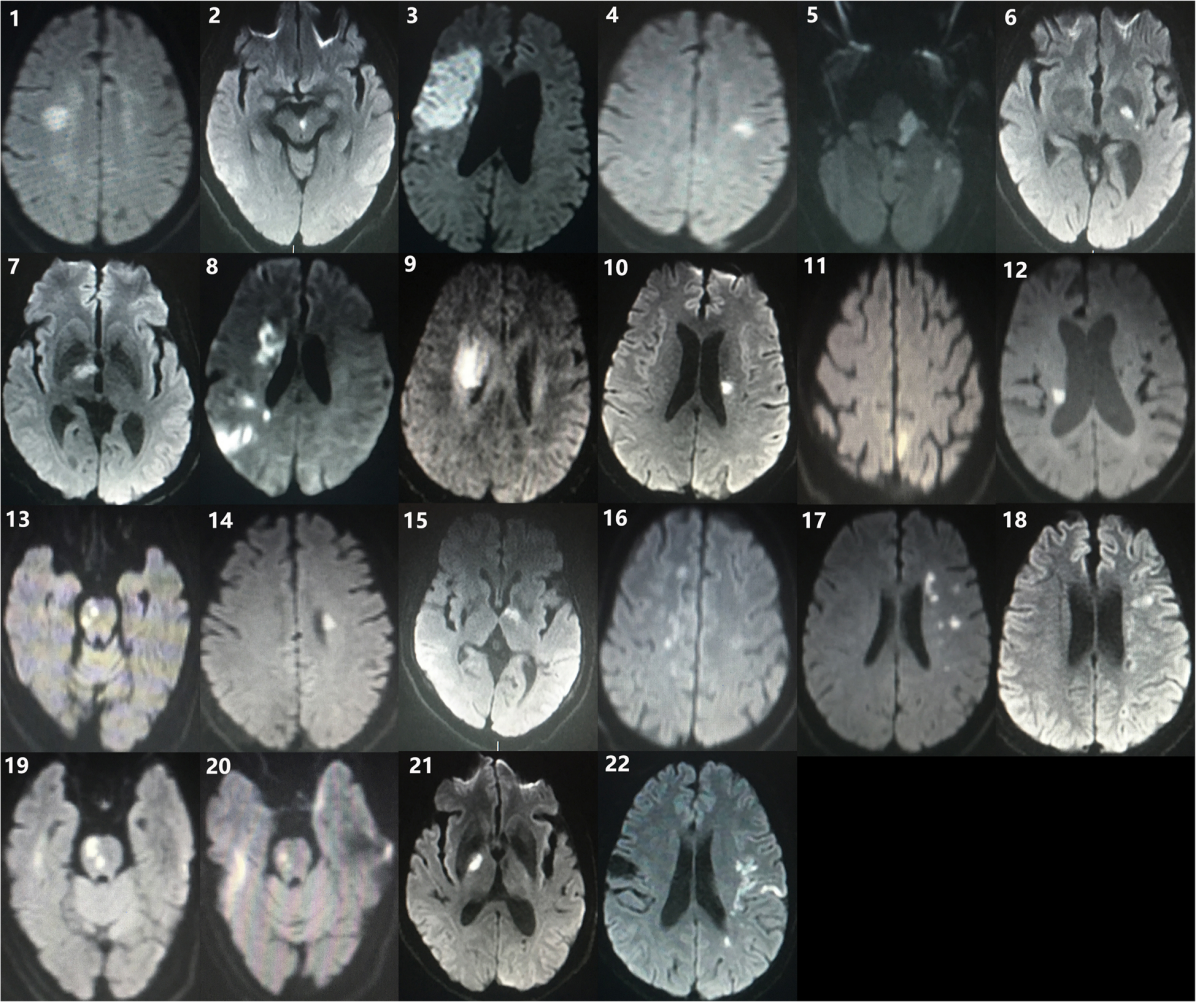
MRI radiographs of patients show responsible lesions of recent stroke on DWI
